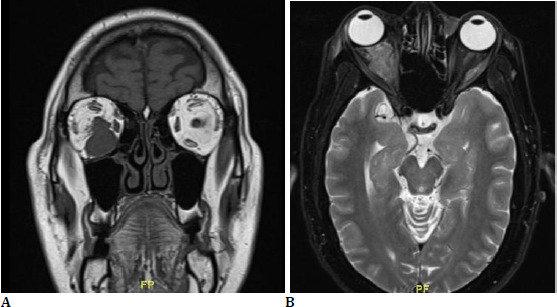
A. T1 MRI coronal section showing a hypointense lesion in the right eye replacing the inferior rectus muscle; B. Axial section showing irregular lesion with 180-degree sectoral contact with optic nerve
